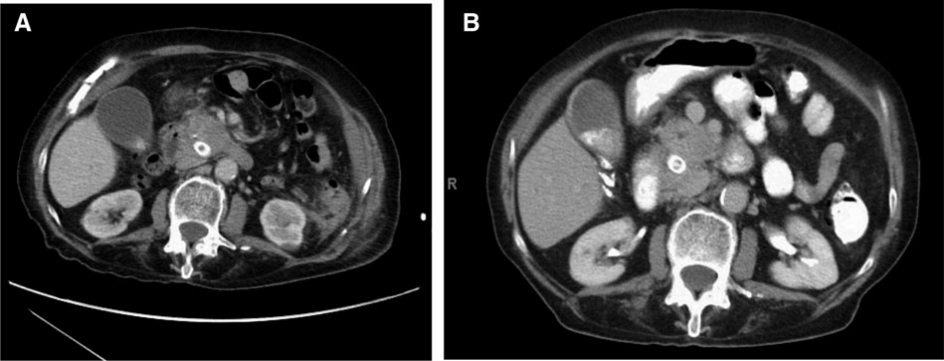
(A) February 2015 CT scan. (B) October 2015 CT scan.
